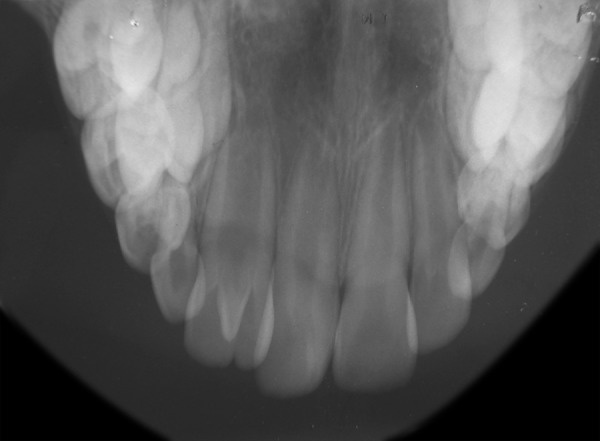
Occlusal radiograph of the maxillary permanent lateral incisors
